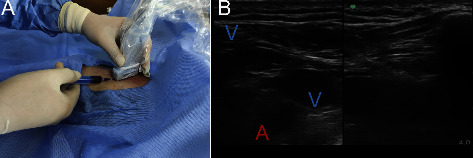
Image and photo of puncturing (A) the real‐time ultrasound image of double screen puncturing. Yellow line shows the needle trajectory. (B) The operating photo of puncturing. “Image and photo of puncturing” the value is in bold format.
